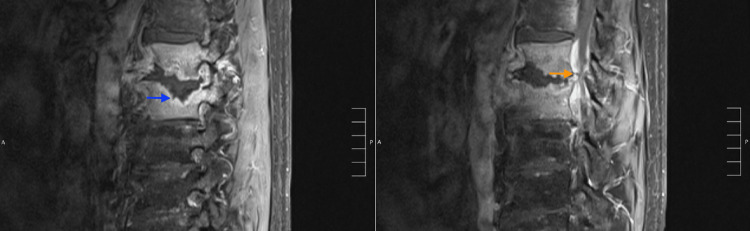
MRI lumbar spine with and without contrast showing findings consistent with advanced discitis, vertebral osteomyelitis, and a compressive epidural abscess at L1/L2 There is erosion of the endplates at L1 and L2 with abnormal edema throughout the vertebral bodies of L1 and L2 and abnormal enhancement within the vertebral bodies. In the disc space there is a rim-enhancing fluid collection and collapsed disc material (blue arrow). At L1 and L2, there is also a circumferential epidural abscess compressing the thecal sac (orange arrow). There is no definite evidence of abnormal enhancement of nerve roots above or below the level of the involvement
